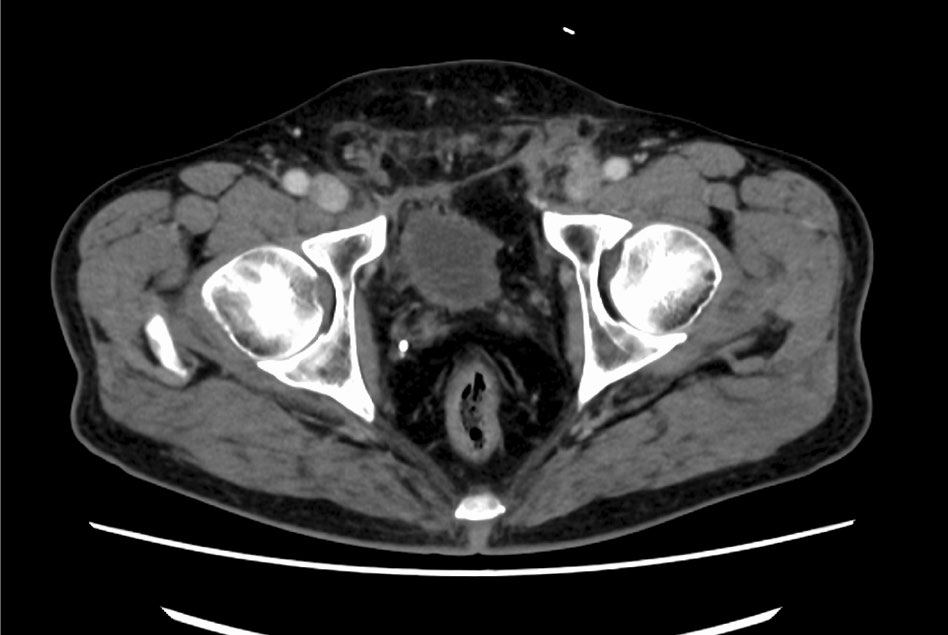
Follow‐up computed tomography scan at 1 month showed that the bladder hernia had disappeared.
